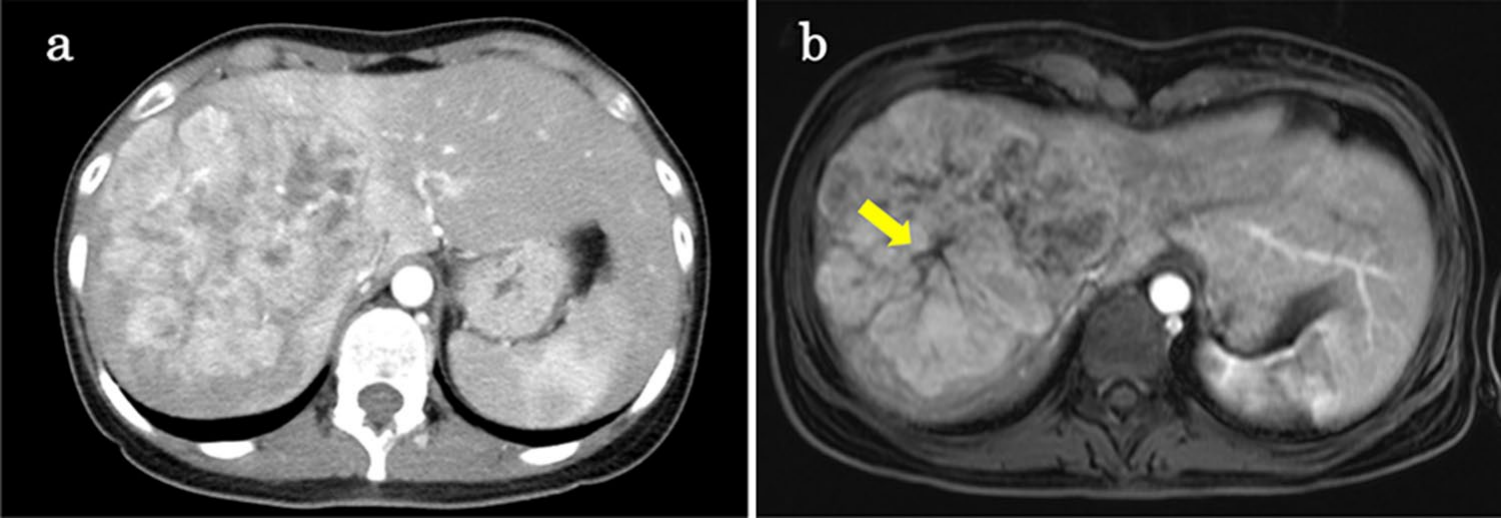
a Dynamic computed tomography shows a 120 mm-sized lobulated tumor occupying the entire right lobe of the liver with heterogeneous enhancement at early phase. b Gadolinium ethoxybenzyl diethylenetriamine pentaacetic acid-enhanced magnetic resonance imaging shows a higher signal tumor compared to the hepatic cells in the early phase, and the center of the tumor resembles scar tissue (arrows)
